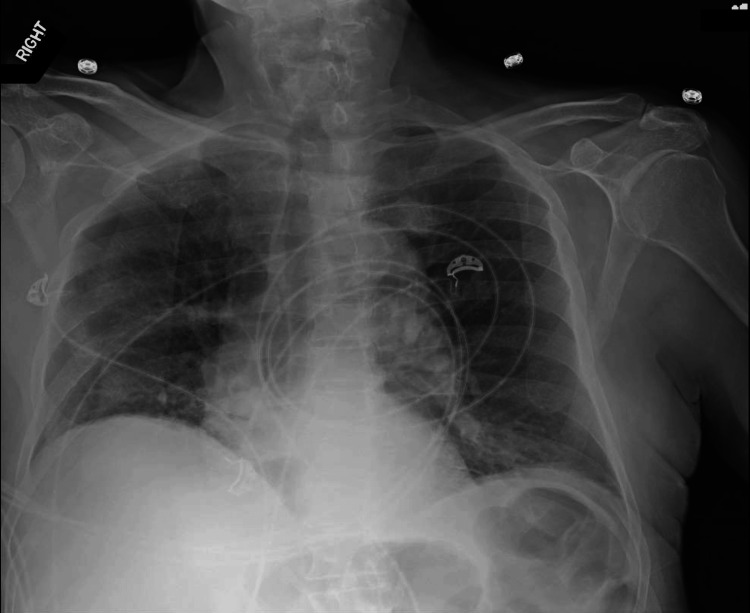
Noncardiogenic pulmonary edema on chest radiograph Chest radiograph demonstrating bilateral pulmonary infiltrates, consistent with noncardiogenic pulmonary edema in the setting of sepsis-related capillary leak
